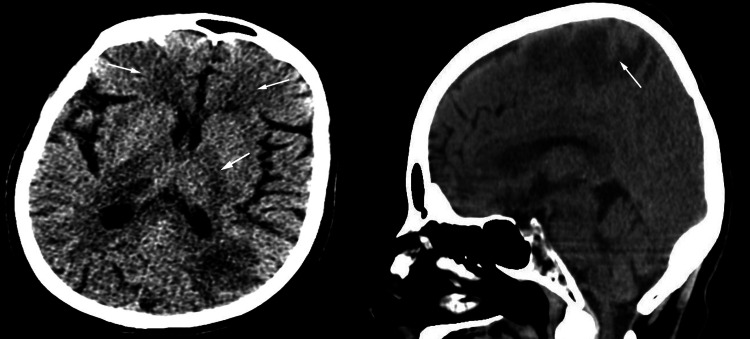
Brain computed tomography (CT) revealed multiple hypodense areas involving the bilateral frontal, right thalamic regions (a), and parietal lobe (b).
